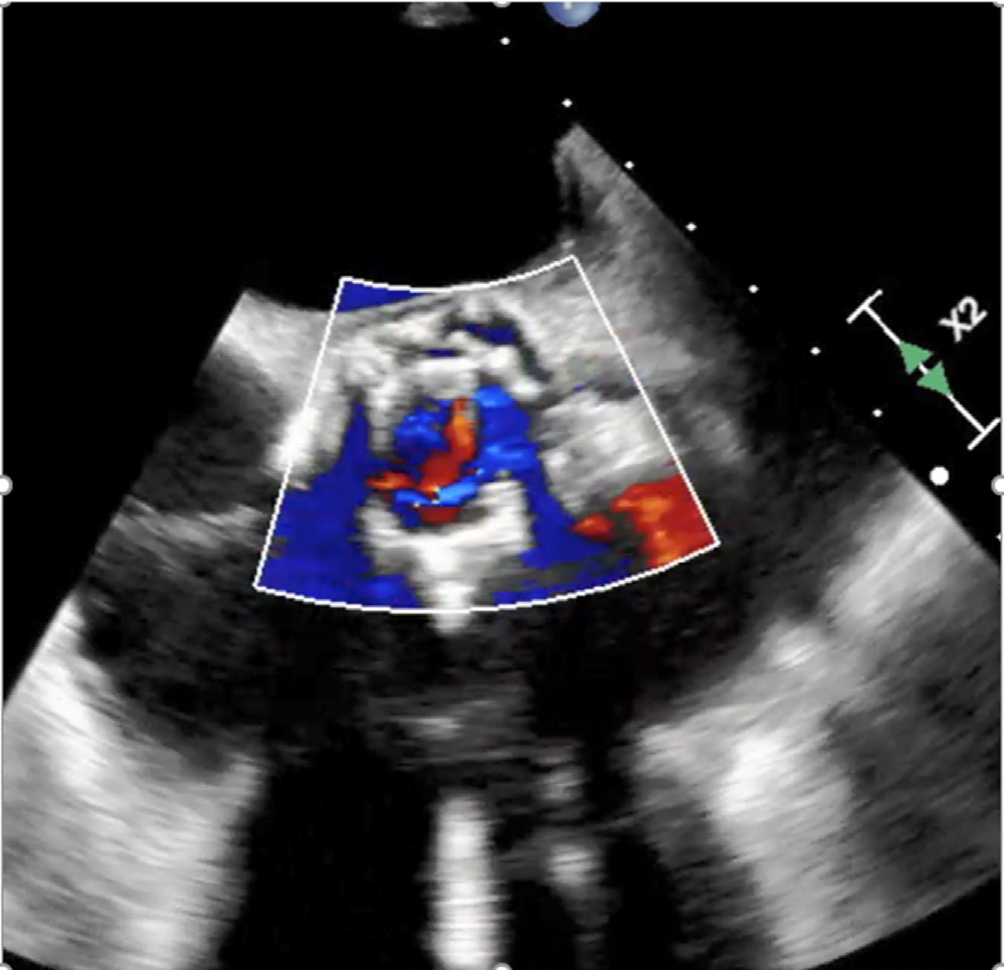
Transesophageal echocardiogram short axis of aortic valve showing resolution of aortic regurgitation.
